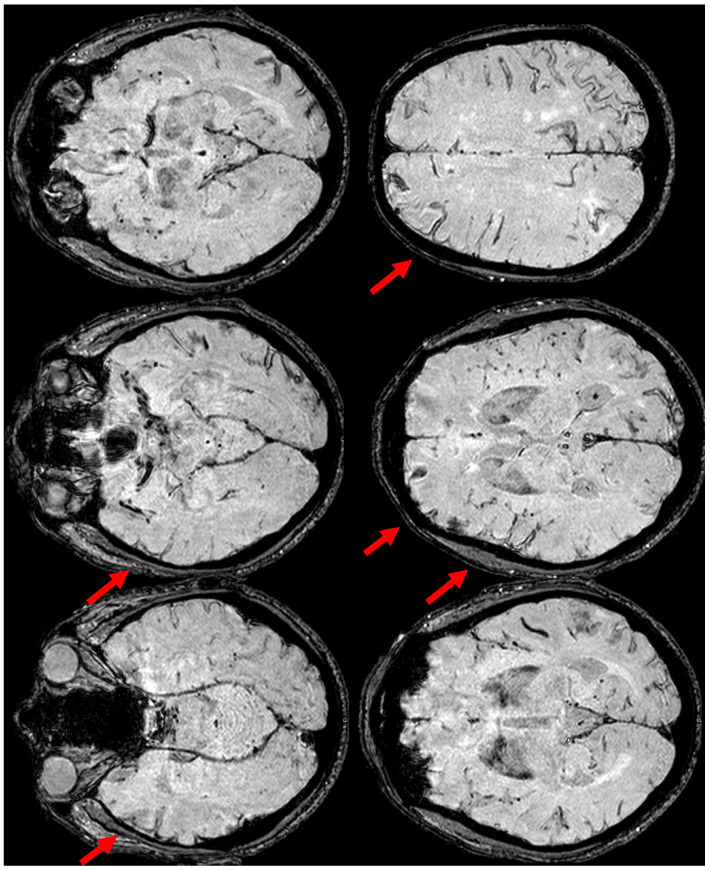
Brain MRI at three months from the start of CAA-related inflammation (Figure 3), showing on axial SWI, the increased extension of cSS, actually involving the left anterior temporal lobe and the left anterior frontal lobe (red arrows). In addition, the involvement of the left frontal sulci is more extensive than in the previous MRI.
